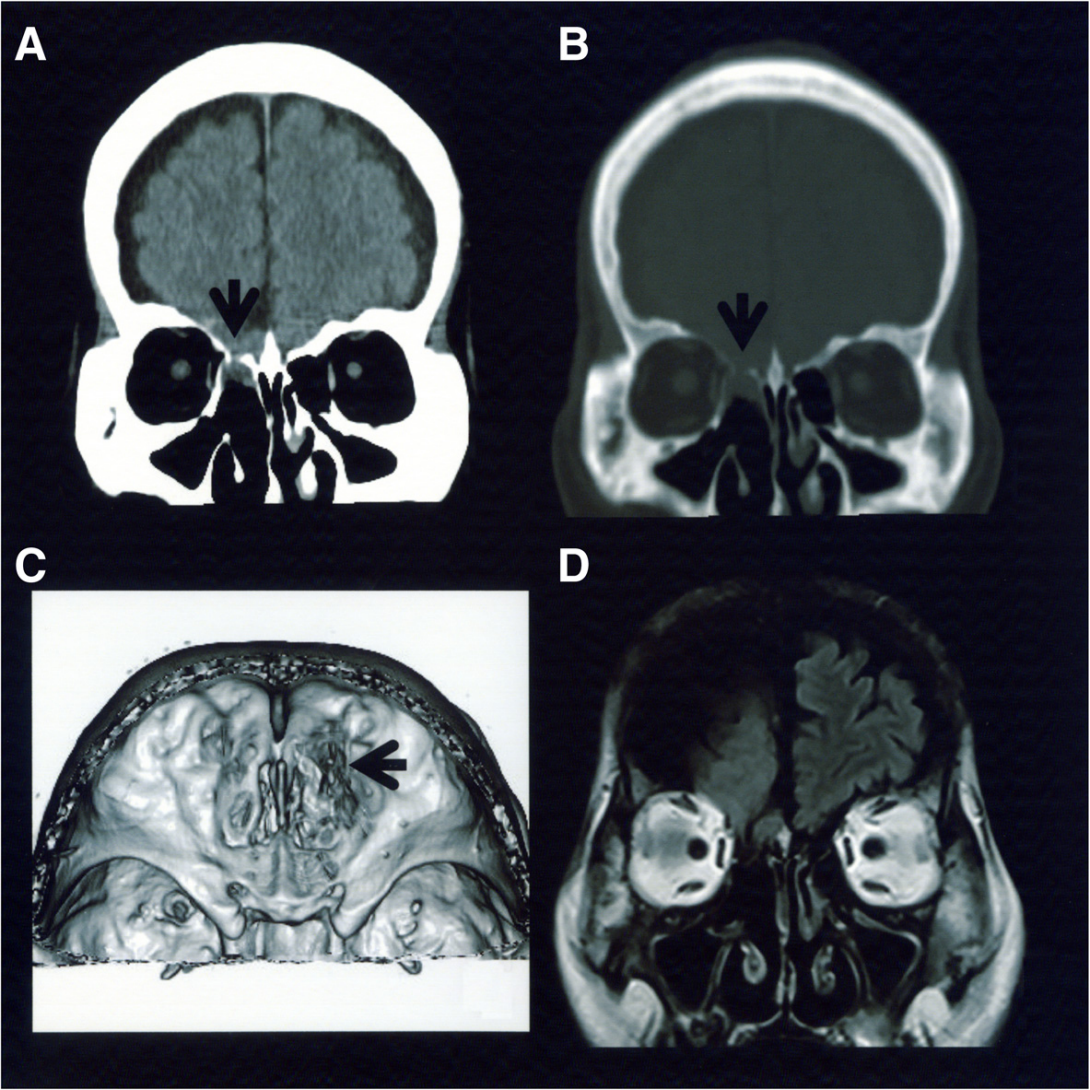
Preoperative neuroradiological examinations. A: Coronal section of computed tomography. An isodense mass protruded through a part of the right ethmoid bone (arrow). B: Bone window computed tomography. A bone defect was noted in the corresponding region (arrow). C: Three-dimensional computed tomography. A round bone defect was present in the ethmoid bone (arrow). D: Magnetic resonance imaging. An isodense mass protruding through the ethmoid bone and a partial cystic component were noted.
